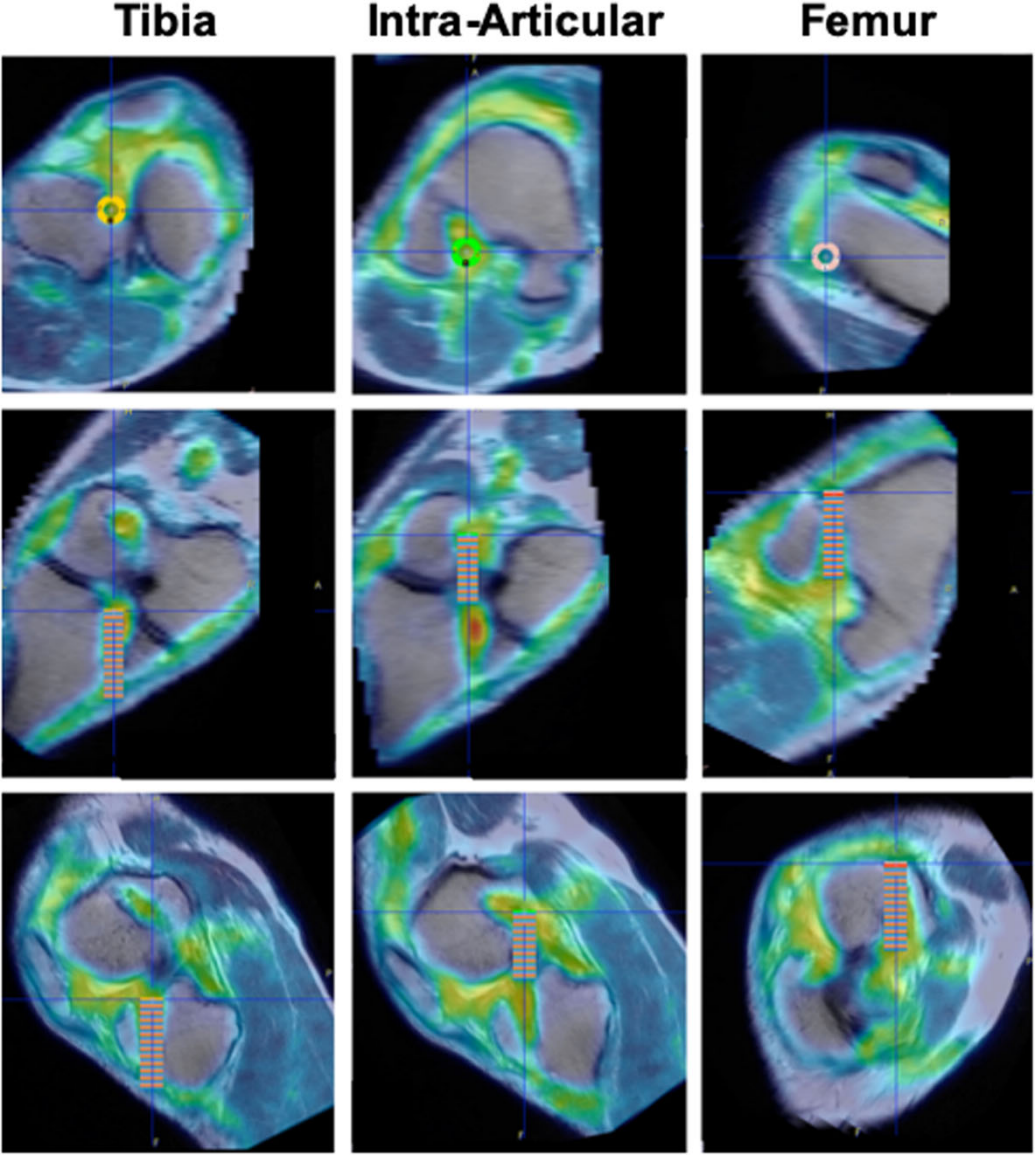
Realignment of PET/MRI images for quantitative analysis. Images showing the re-sliced PET/MRI images for the tibial tunnel, intra-articular graft, and femoral tunnel. The images were aligned so that the tunnels and graft were perpendicular to the imaging plane on axial (top), sagittal (middle), and coronal (bottom) views. Multiple circular regions of interest matching the tunnel diameters were then positioned on the images (colored overlay) to quantify regional ^18^F-FDG uptake
